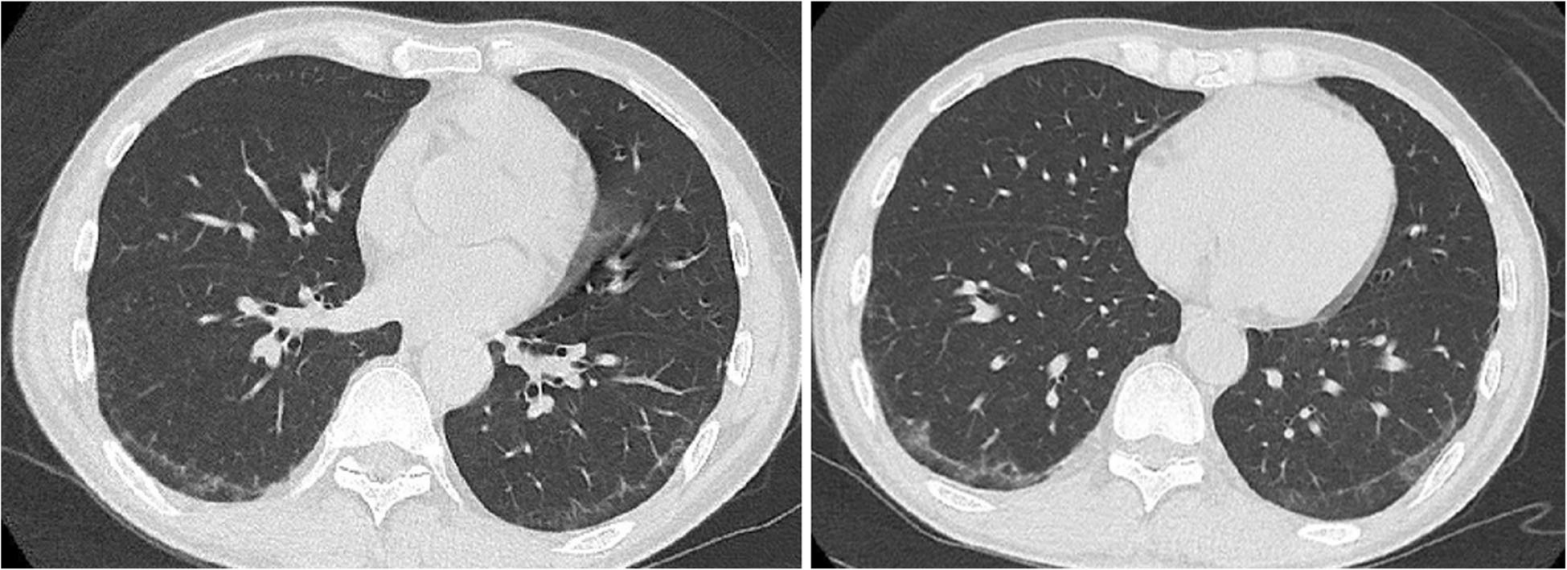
Chest CT scan showed interstitial change in both lower lungs under the pleur without active TB lesion
